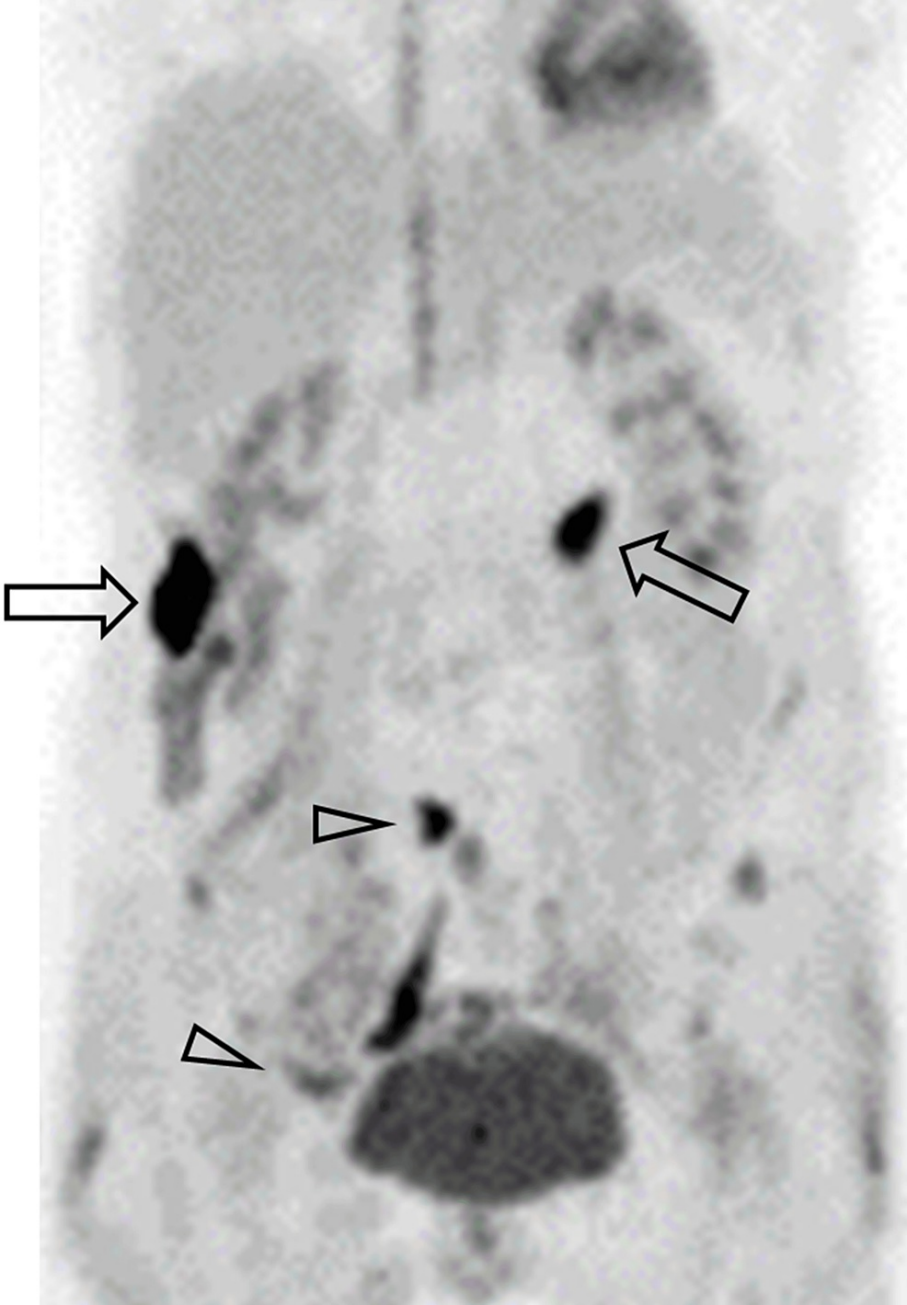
PET/CT PET/CT shows two positive spots in the ascending colon and transverse colon (arrows) and light positive spots in the pelvic area (arrowheads). PET/CT: positron emission tomography/computed tomography
